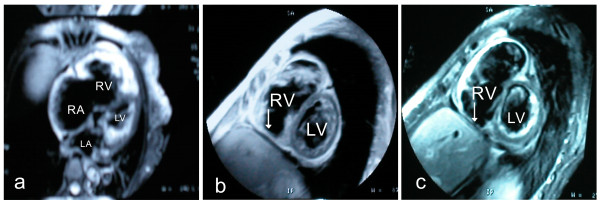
a: The cardiac MRI showed enlargement of right ventricle and atrium without enlargement of left ventricle and atrium; b: the thickness of right ventricle wall and interventricular septum was uneven; high signals indicated fatty tissue replacement (arrows); c: the signals in right ventricule free wall discontinued after inhibiting fatty tissues (arrows).
